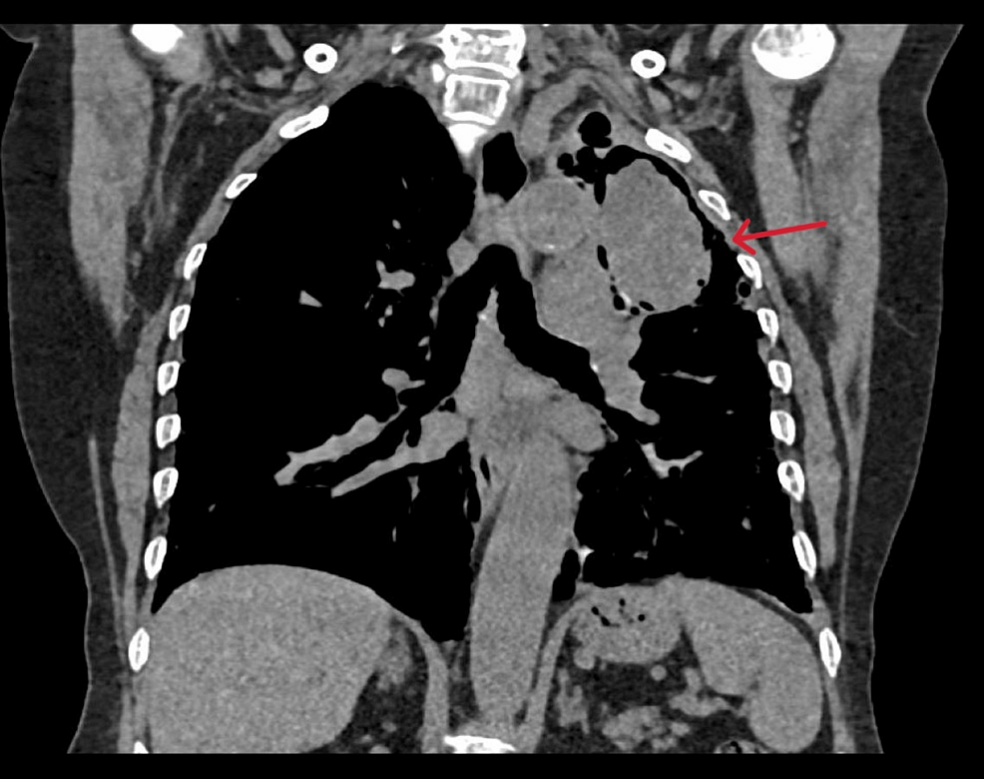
Computed tomography of the thorax-mediastinal window; coronal section showing a large tissue density lesion in the left upper lobe.
